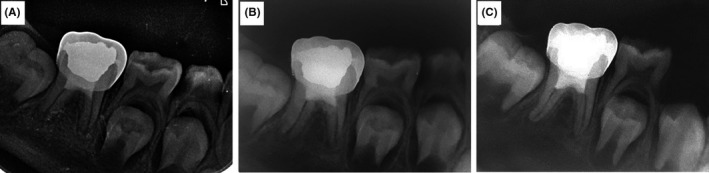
Follow‐up radiographs after revascularization. (A) 3‐mo, (B) 6‐mo, and (C) 12‐mo which show complete root development and healing of periapical lesion
